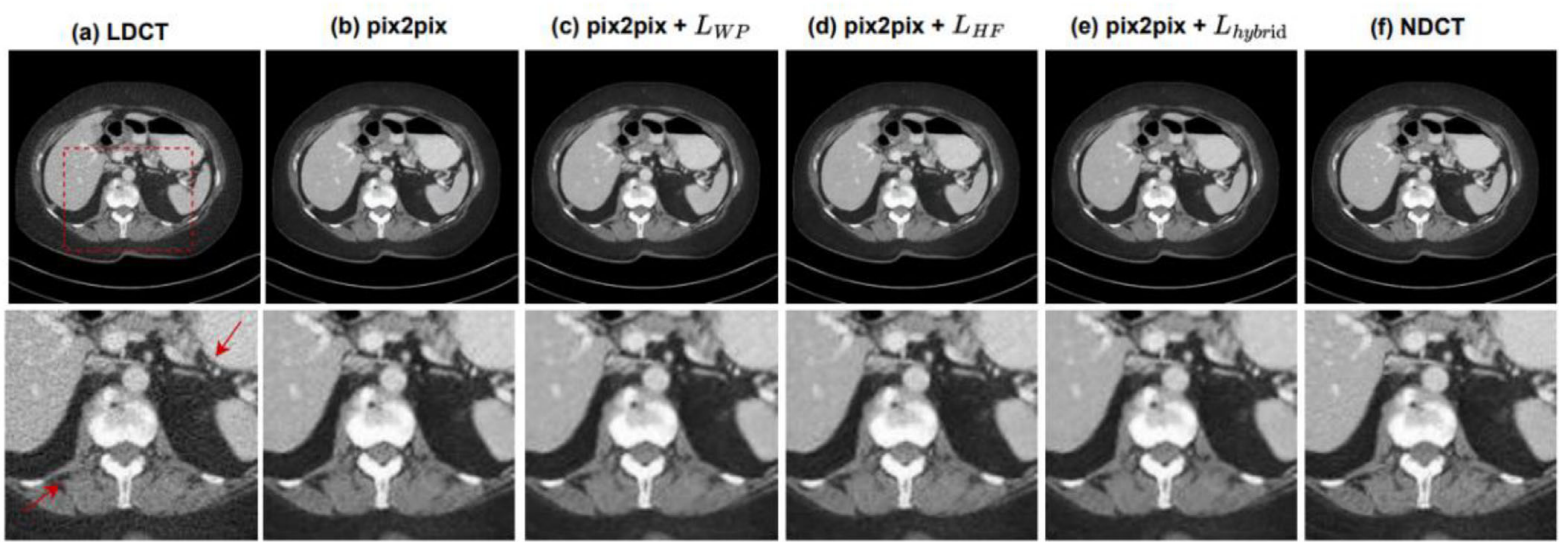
Denoising performance comparison of pix2pix with different loss functions for the abdominal slice in the testing dataset. (a)LDCT, (b)pix2pix with original loss function, (c)adding WPLoss, (d)adding HFLoss, and (e)adding hybrid loss function denoised results, (f)NDCT. The CT display window for the images is [−160 240] HU. The results show that both WPLoss and HFLoss can improve the denoising effect of pix2pix, and when using the hybrid loss, the generated image is the closest to NDCT.
